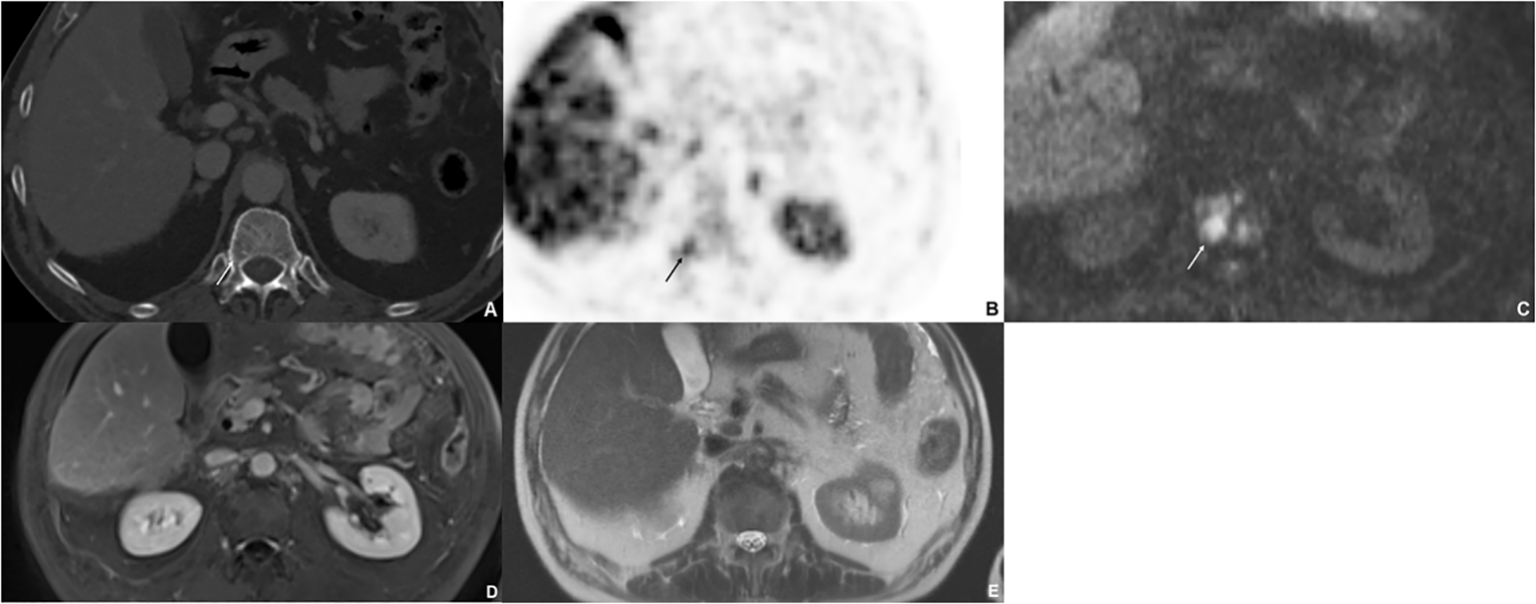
Sixty-nine-year-old male with bone metastases of gastrointestinal NET. The CT (A) shows a lytic lesion (arrow) that is difficult to define; however, the bone metastasis shows a significant uptake ^18^F-SIFA-TATE PET/CT (B). The lesion is strongly diffusion restricted (C); however, it was not visible on postcontrast T1w image (D) nor on T2w image (E).
